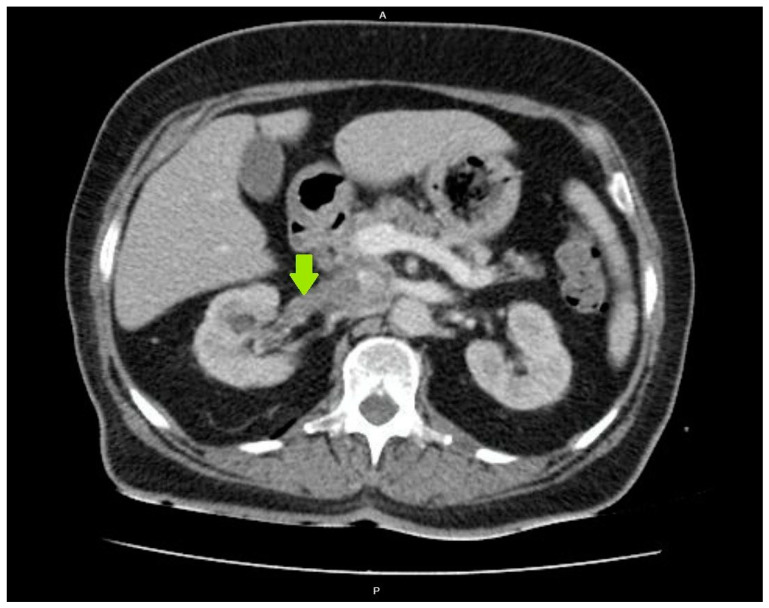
Renal vein thrombosis on a computed tomography (CT) scan of the abdomen.
